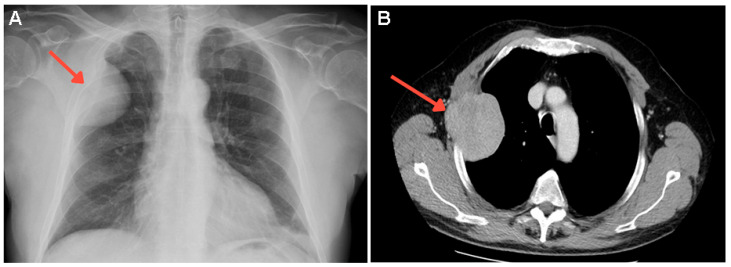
Almost 4 years after initial acromegaly diagnosis, the subject experienced a rapidly growing, painful lump in the right lateral thoracic area. X-ray identified a large, nodular opacity of 7.3 cm, located in the right upper latero-thoracic chest wall (red arrow) (A). Thoracic contrast-enhanced computed tomography (CECT) revealed an osteolytic lesion of 9 cm (red arrow) invading the third right rib (B). At that point, he was undergoing lanreotide 120 mg every 42 days for 6 months. The endocrine assessment showed normal IGF-1, but the nadir of OGTT-GH was 1.29 ng/mL, suggesting suboptimal disease control. Otherwise, the biochemical and hormonal panel was normal, including total serum calcium, total alkaline phosphatase, renal function, and parathyroid hormone (PTH) (Table S1).
